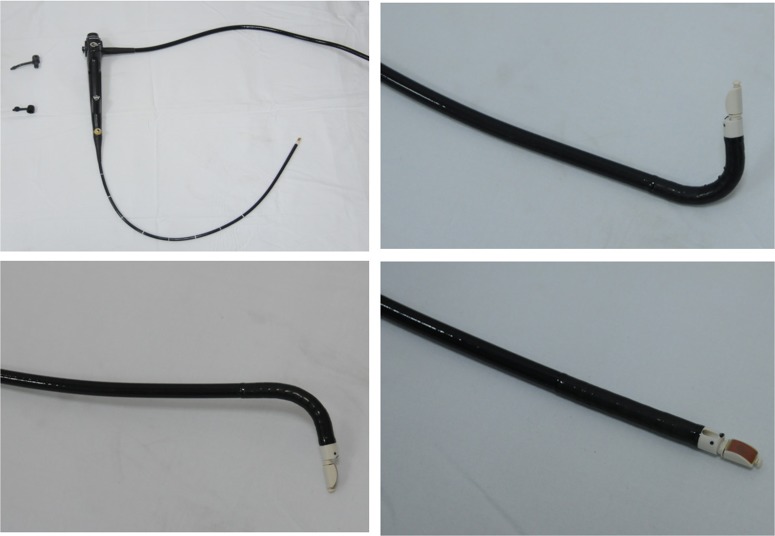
The flexible ultrasound bronchoscope.
